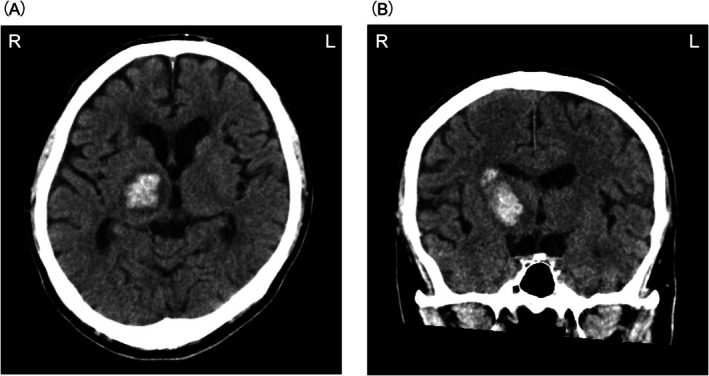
Computed tomography images of the brain at admission. (A) Horizontal plane. (B) Frontal plane. Hemorrhage in the right thalamus.
